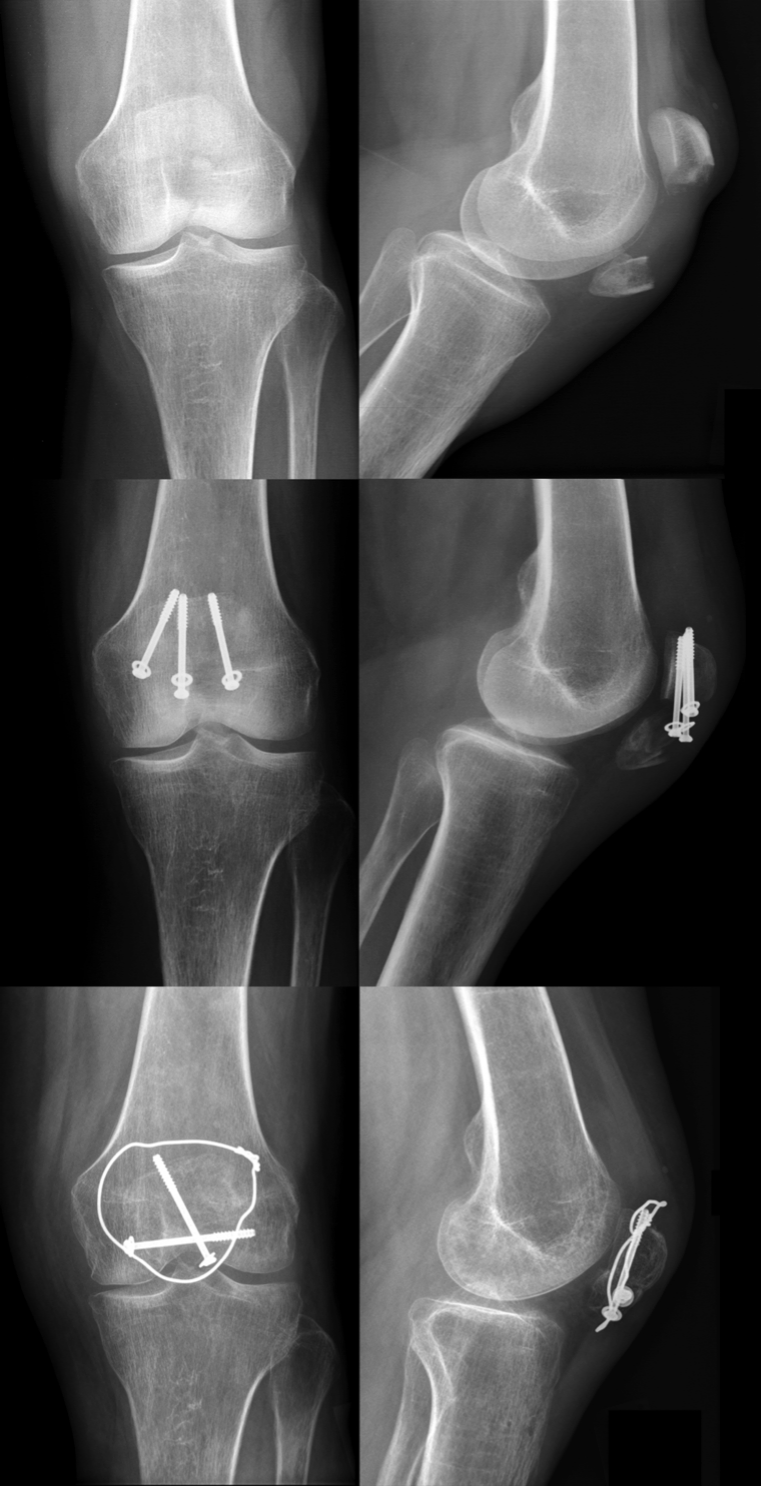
Preoperative X-rays of a transverse patella fracture (above). Loss of reduction with screw pullout 3 month after surgery (middle). 3 months after revision surgery with screw fixation and additional modified tension band (below).
